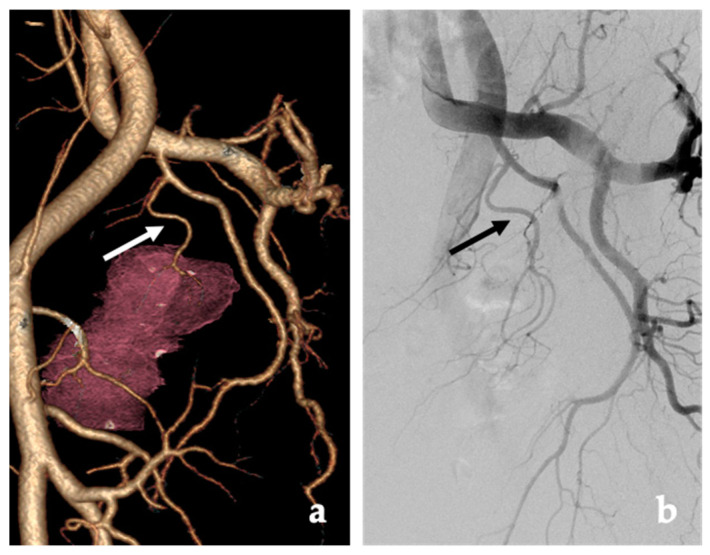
Computed Tomography Angiography (CTA) is useful for PAE procedural planning. (a) CTA 3-D reconstruction using the dedicated prostate artery protocol demonstrates the prostate artery origin arising from the internal pudendal artery (white arrow). (b) Digital subtraction angiography (DSA) imaging performed at 35 degrees LAO projection shows the prostatic artery arising from the internal pudendal artery (black arrow) correlating with the CTA.
